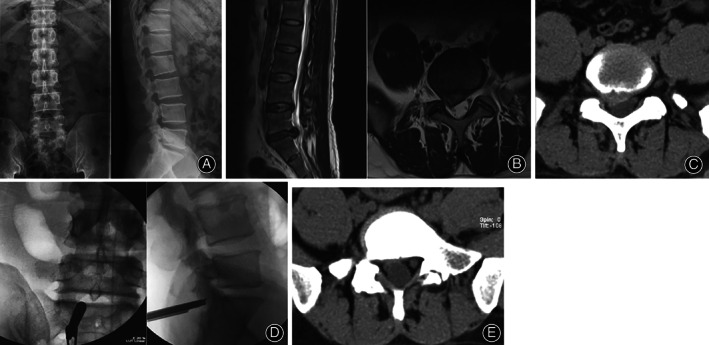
Male, 42 years old. Main complaint: lumbago for 3 years, with pain and numbness of right lower extremity for 1 month. (A) Preoperative X‐ray films. (B) Preoperative MRI showed L_5_S_1_ disc herniation. (C) Preoperative CT showed L_5_S_1_ disc herniation. (D) The position of the working cannula through the inter lamina approach during the operation. (E) CT image after operation shows that the herniated intervertebral disc has been taken out.
